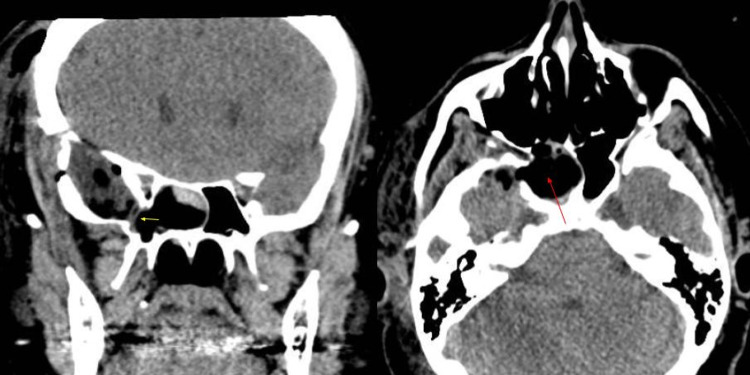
Head computed tomographic scan after surgical correction via a right pterional craniotomy with postsurgical changes in the anterior temporal lobe and a small amount of pneumocephalus. Fat graft has been placed inside the right sphenoid sinus (red arrow). The defect had been covered with a collagen dural substitute (yellow arrow) Coronal (left), axial (right)
